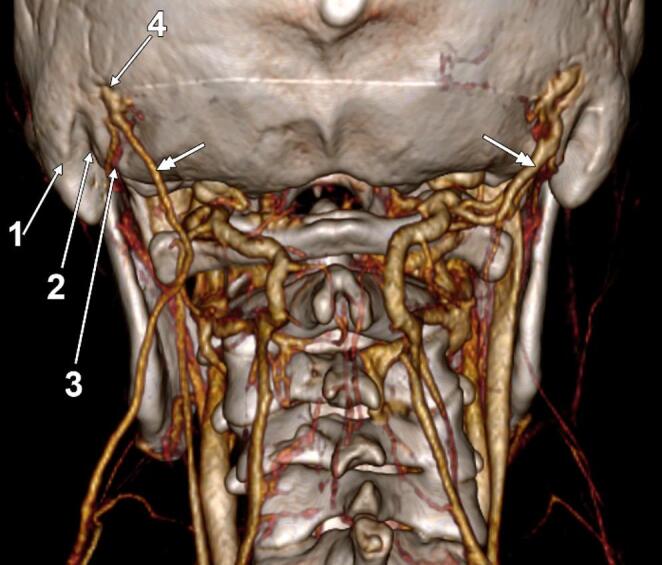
Bilateral mastoid emissary veins (double-headed arrows). CT angiogram, three-dimensional volume rendering. Posterior-inferior view. 1. mastoid process; 2. mastoid/digastric notch; 3. occipital artery sulcus; 4. mastoid foramen
